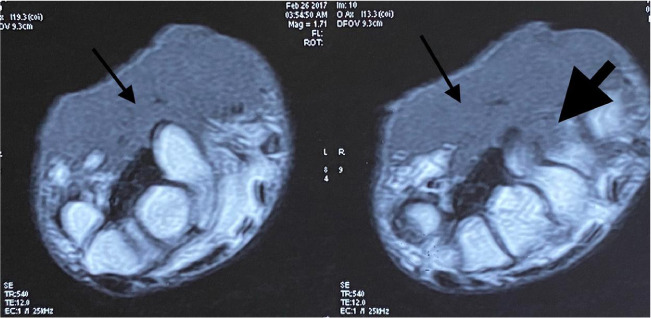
MRI images of the right wrist- (T1WI axial) showing an ill-defined soft tissue lesion involving the flexor aspect of the distal third of the forearm and wrist (thin black arrow). Lesion involving the pisiform bone (thick black arrow).
